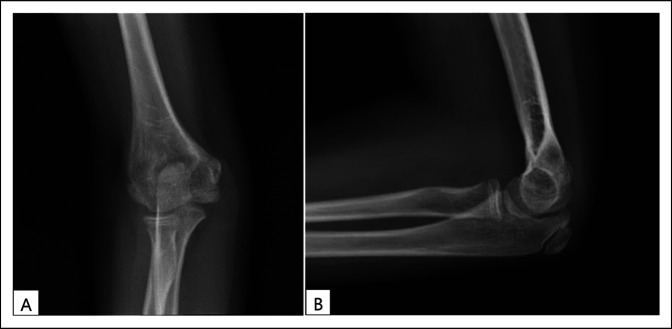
Follow-up radiographs of the right elbow at 5 years. A, Anteroposterior view. B. Lateral view.
